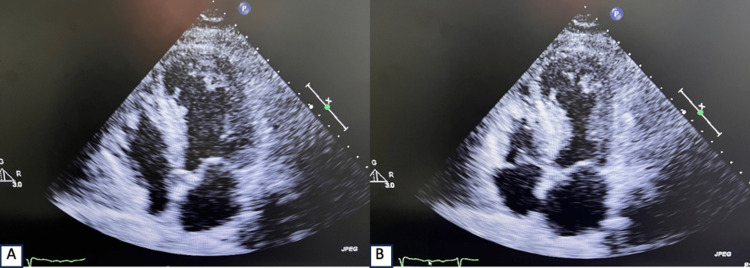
Panel A and B: TTE apical four-chamber view showing basal hyperkinesis with apical ballooning
